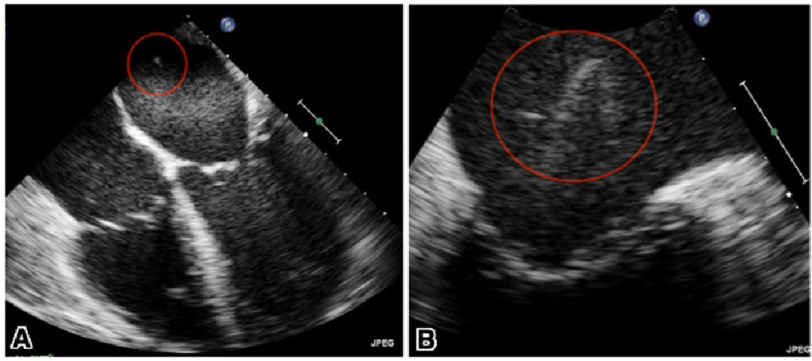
Transesophageal echocardiography showing a long, filamentous, highly mobile mass attached to the posterior wall of the left atrium (A). Magnified image demonstrating the mass measuring 1.7 cm length and 2 mm width (B).
